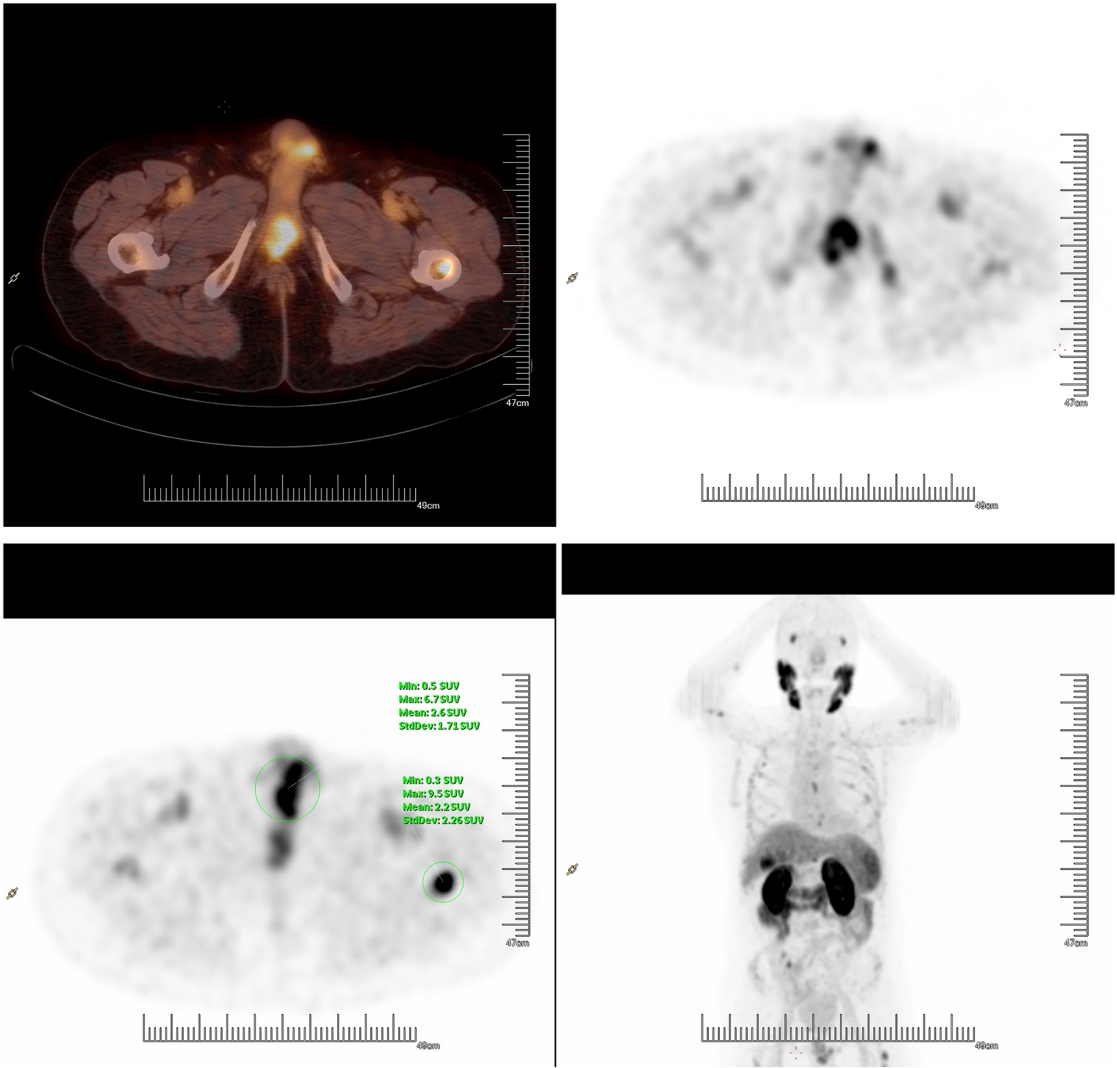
^18^F prostate-specific membrane antigen PET/CT showed suspicious tracer uptake in the base of the penis and multiple bone metastases.
